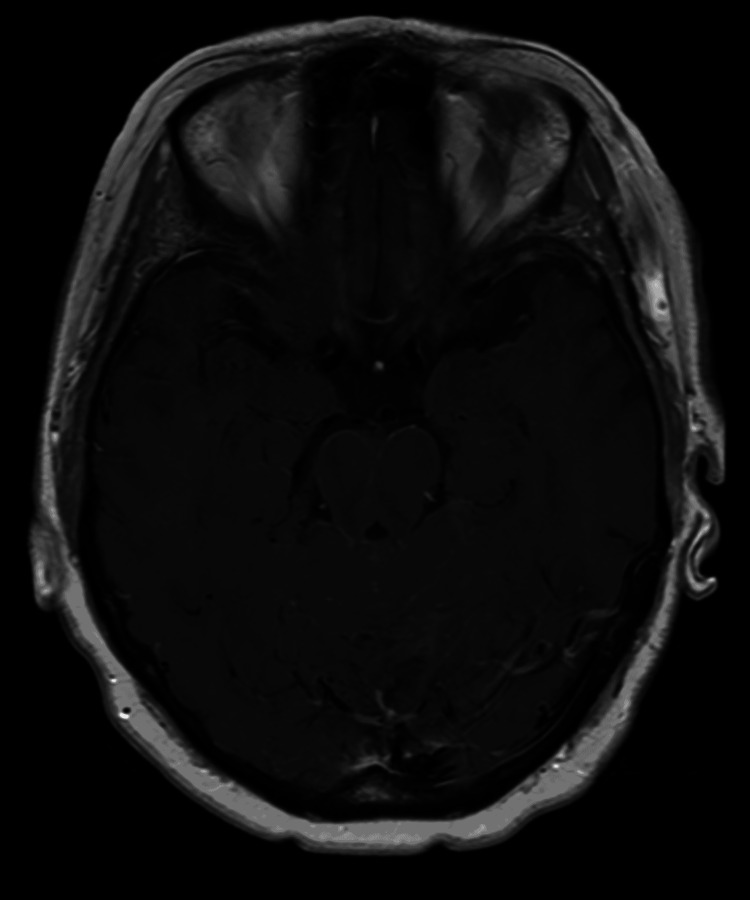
Brain MRI axial T1 post-contrast 12 days prior to admission. MRI: magnetic resonance imaging
